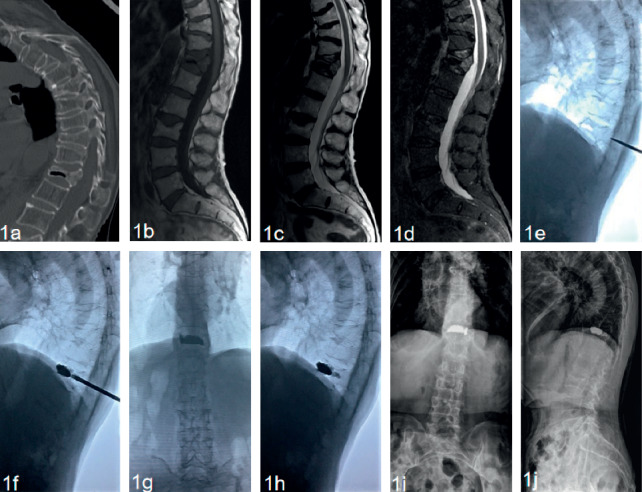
A 78-year-old male patient with Kümmell's disease at T12 treated by PVP. (a) Preoperative sagittal CT scan showed the intravertebral vacuum sign; (b) sagittal T1-weighted MR image showed a low signal intensity in the location of the cleft; (c, d) sagittal T2-weighted MR image and short tau inversion recovery (STIR) image showed a well-defined low signal intensity in the location of the cleft; (e) the height of the affected vertebral body was partly recovered at a hyperextension position; (f) the low-viscosity PMMA adhered to the previous high-viscosity mass and diffused into the intervertebral trabecular space; g-h: X-ray immediately after operation showed the bone cement filled the cleft without leakage. The symmetric distribution of the bone cement was verified (g), the low-viscosity PMMA was firmly fixed in the vertebral body like a ship anchor to prevent the slide of the highviscosity mass of bone cement (h); (i, j) no delayed displacement of bone cement, or adjacent vertebral fracture were observed at the last follow-up.
